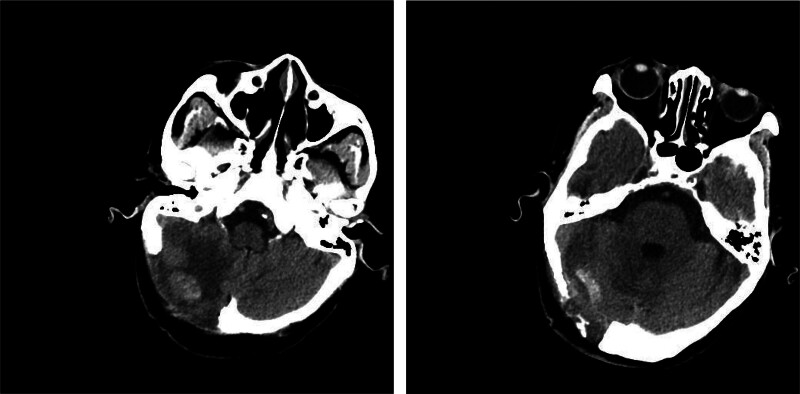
CT image of the head on the first postoperative day. CT = computed tomography.
